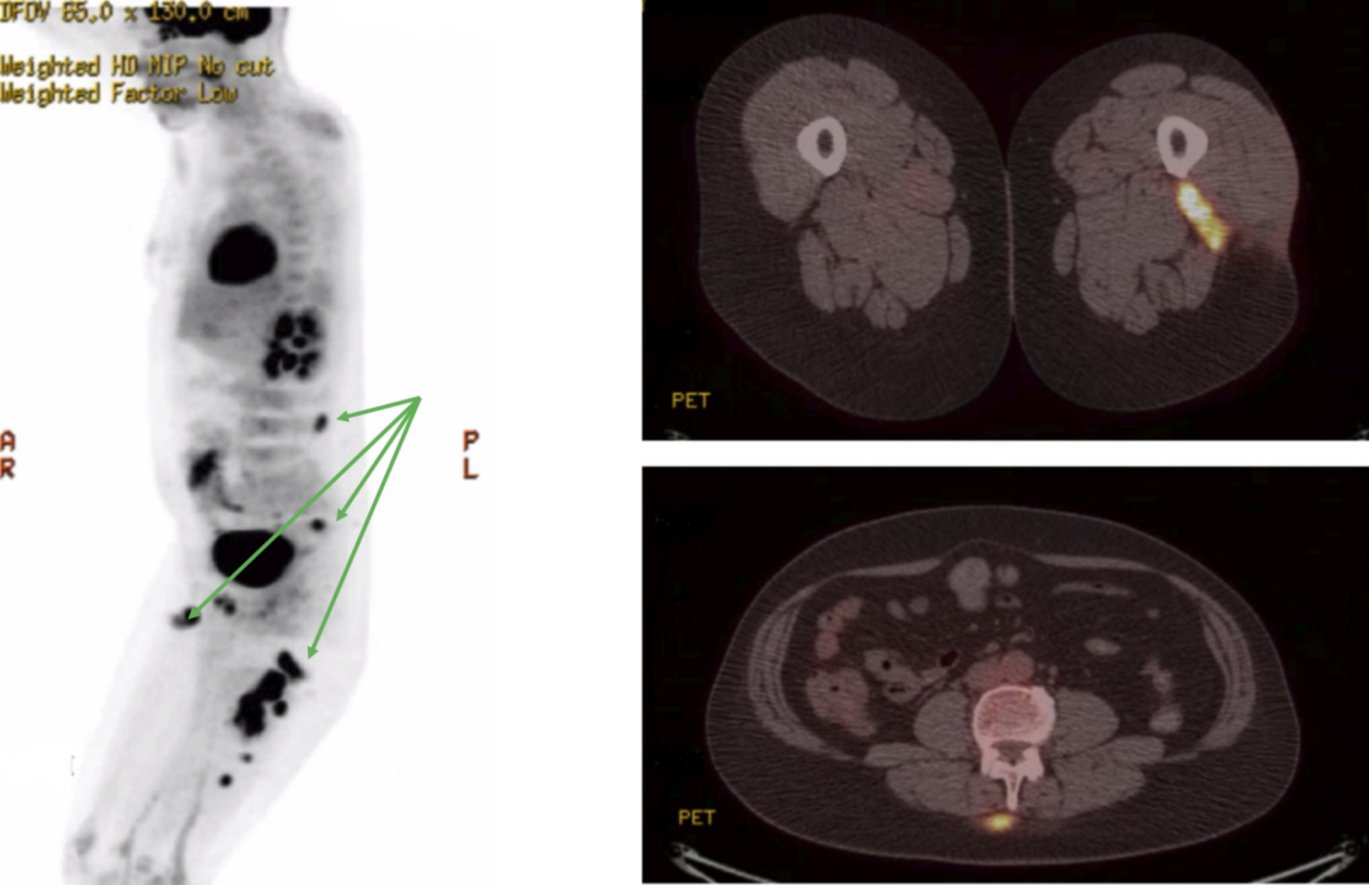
Imaging depicts multiple subcutaneous soft tissue nodules noted in the lower back at the level of L4 and in the bilateral thighs demonstrating increased fluorodeoxyglucose (FDG) uptake suggestive of cutaneous extranodal Rosai-Dorfman disease
